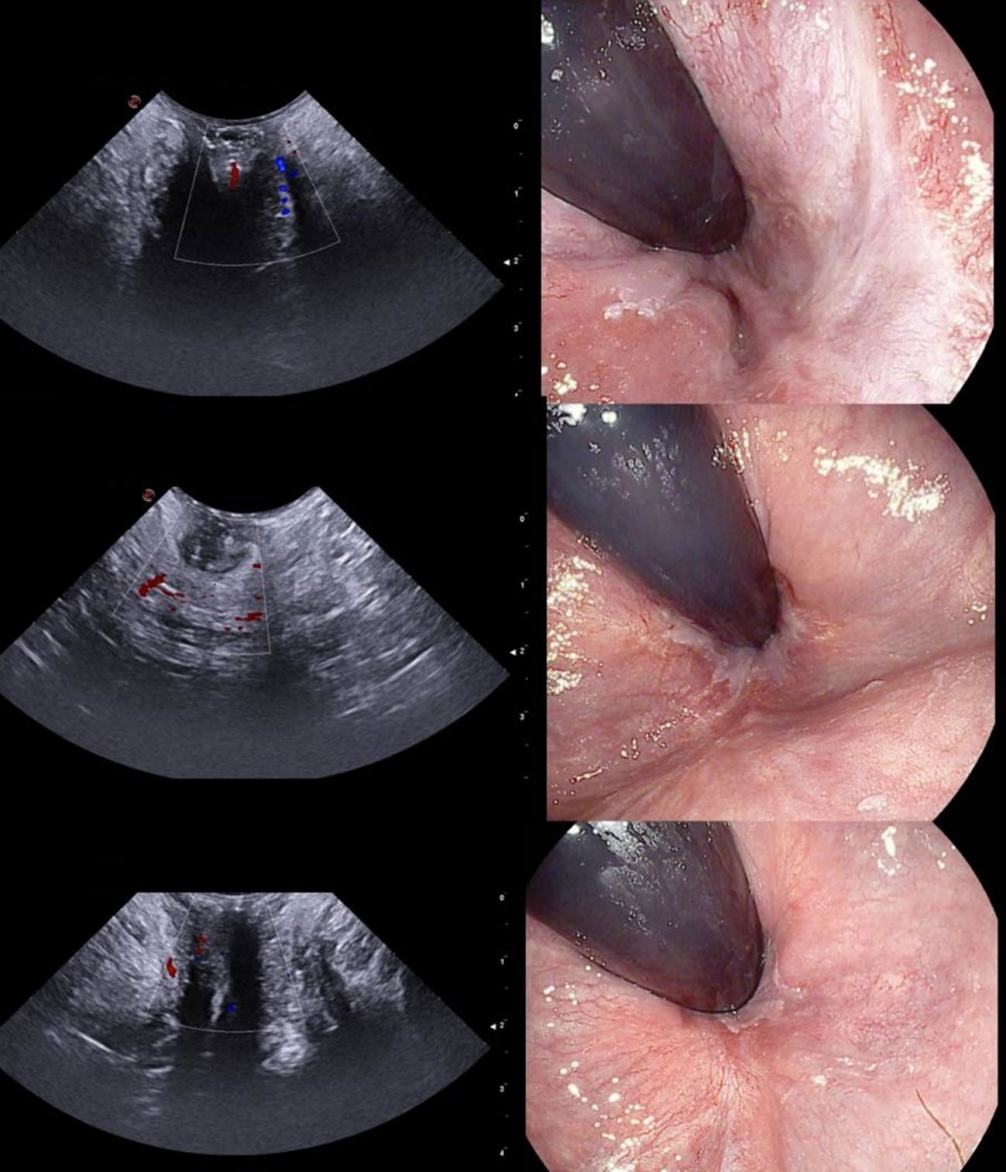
Comparison of the scattered pattern on transperineal US Doppler (left panels) with corresponding endoscopic images (*right panels*)
